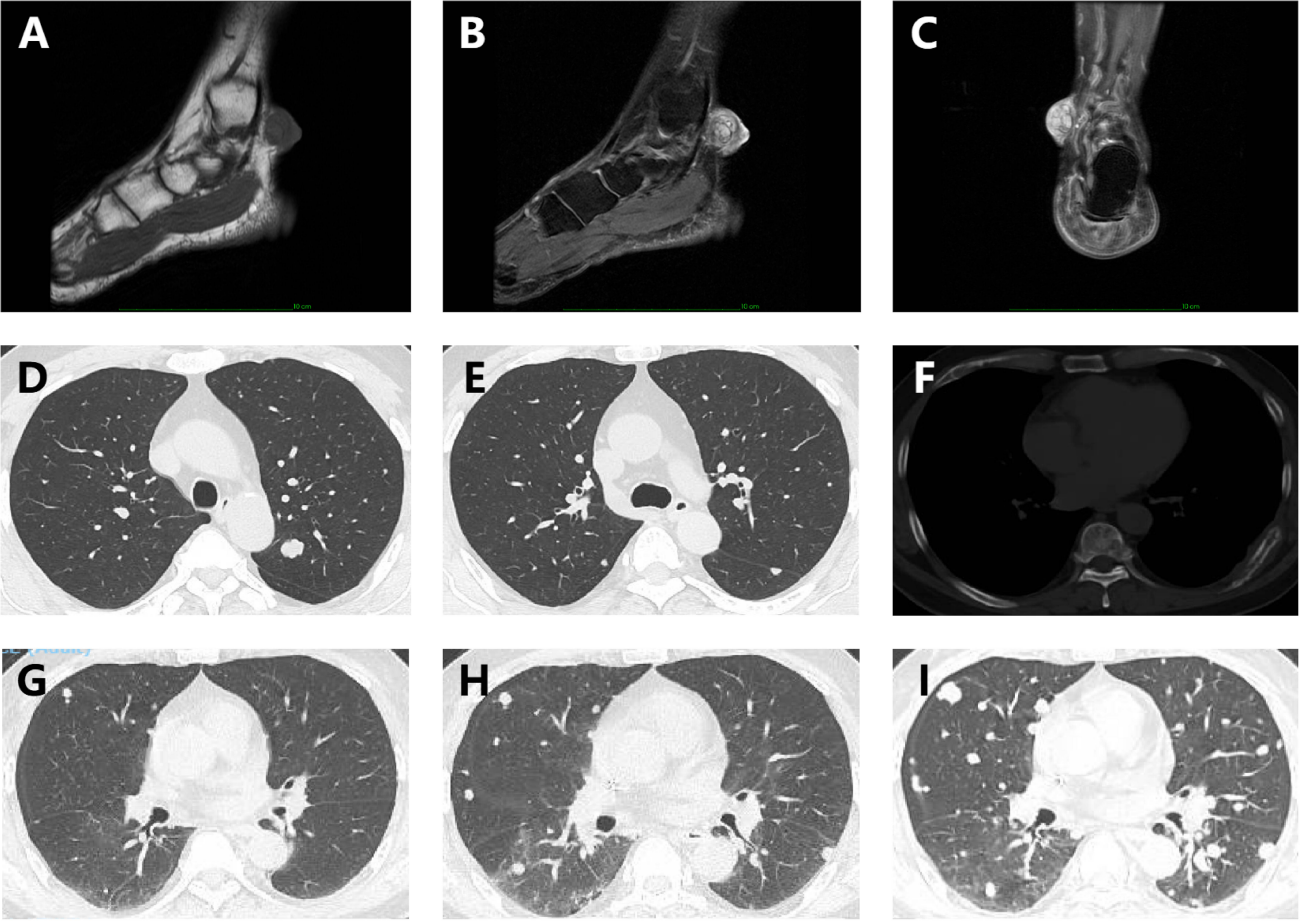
Initial MRI of the left ankle at disease onset and serial follow-up chest CT images. (A-C) Magnetic Resonance Imaging (MRI) revealed a well-defined, round mass located within the subcutaneous fat layer of the medial aspect of the left posterior ankle. On sagittal T1-weighted imaging, the lesion appears isointense; On sagittal and coronal fat-suppressed T2-weighted imaging, the lesion demonstrates heterogeneous hyperintensity with internal linear hypointense strands. (D-F) Chest CT performed on August 3, 2021, demonstrated multiple metastatic lesions in both lungs as well as a metastasis in the left eighth rib. (G) Chest CT on January 10, 2022; (H) Chest CT on March 9, 2022; (I) Chest CT on May 26, 2022.
